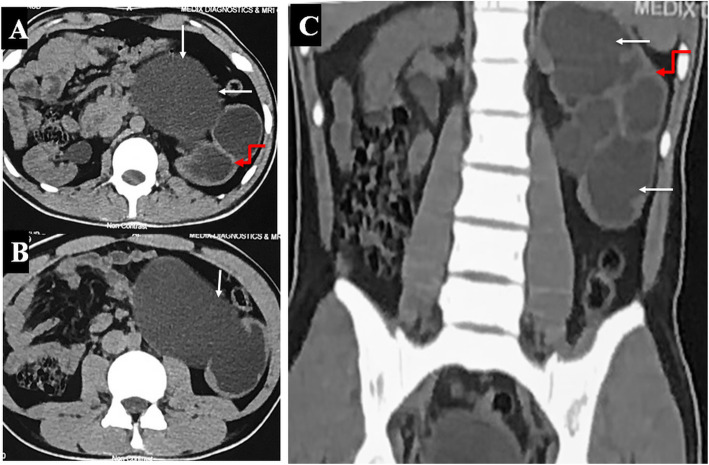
Axial (a, b) and coronal (c) non-contrast-enhanced CT (NCCT) scan images showing gross hydronephrosis of the left kidney (white arrows) with severe parenchymal thinning (curved red arrows)
